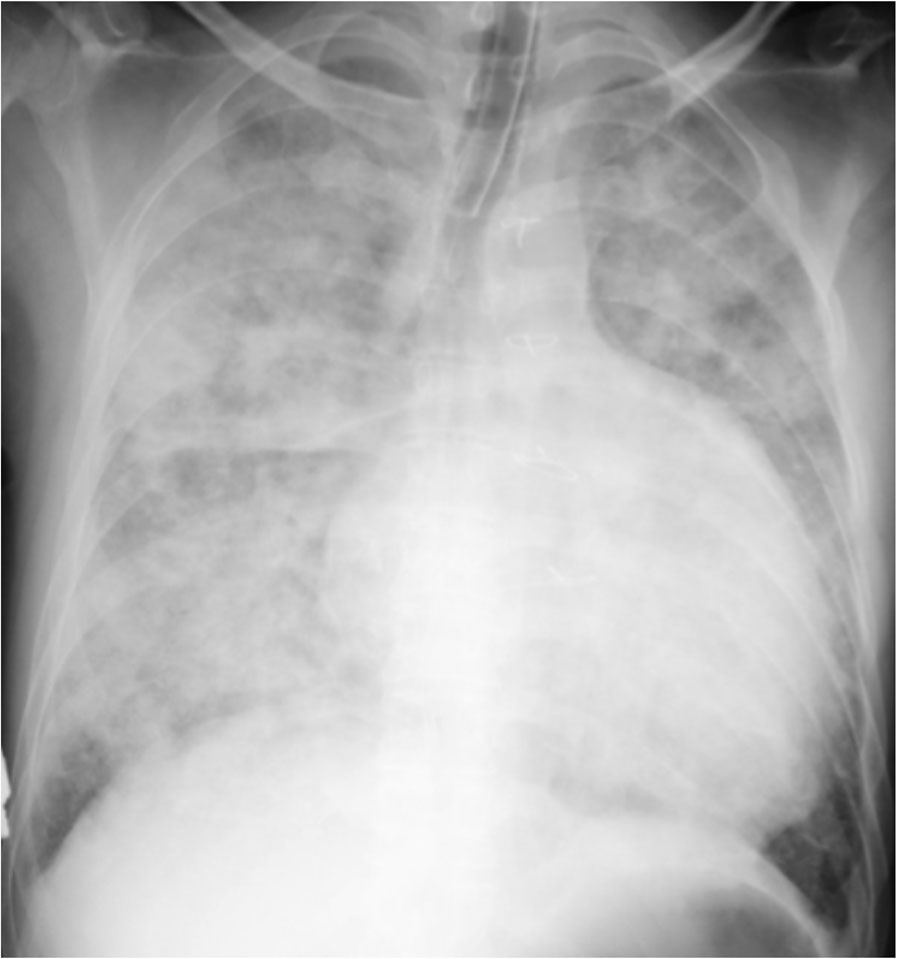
Chest radiography shows pulmonary edema and an extremely dilated ventricular shadow at the onset of severe right ventricular failure
